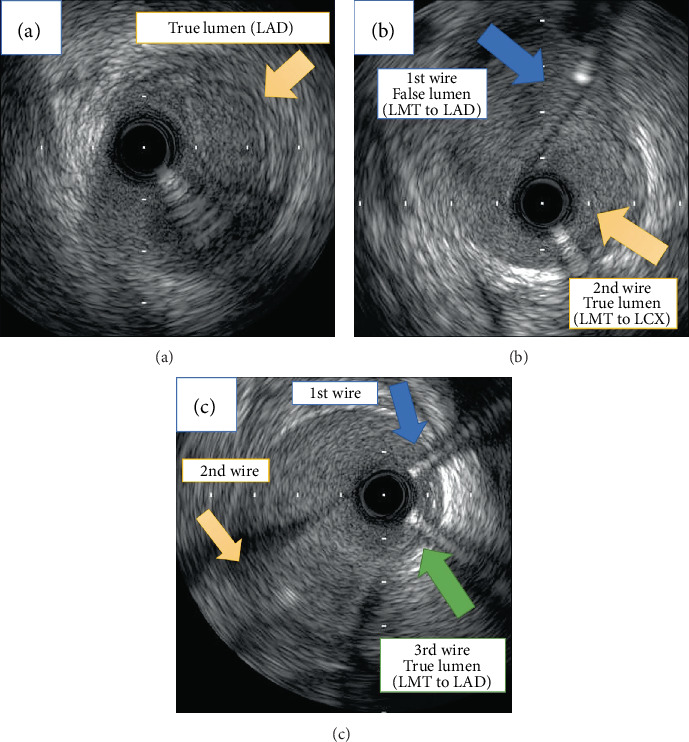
IVUS findings during percutaneous coronary intervention. (a) The first guide wire passed through the false lumen from the midportion of the LMT to the proximal site of the LAD, and the true lumen of the LAD was compressed by the enlarged false lumen (orange arrow). (b) The second guide wire was inserted into the LCX, and IVUS imaging confirmed that it had been placed in the true lumen from the LMT to LCX (yellow arrow). (c) The third guide wire passed entirely through the true lumen from the LMT to LAD (green arrow). LMT: left main trunk; LAD: left anterior descending artery; LCX: left circumflex artery; IVUS: intravascular ultrasound.
